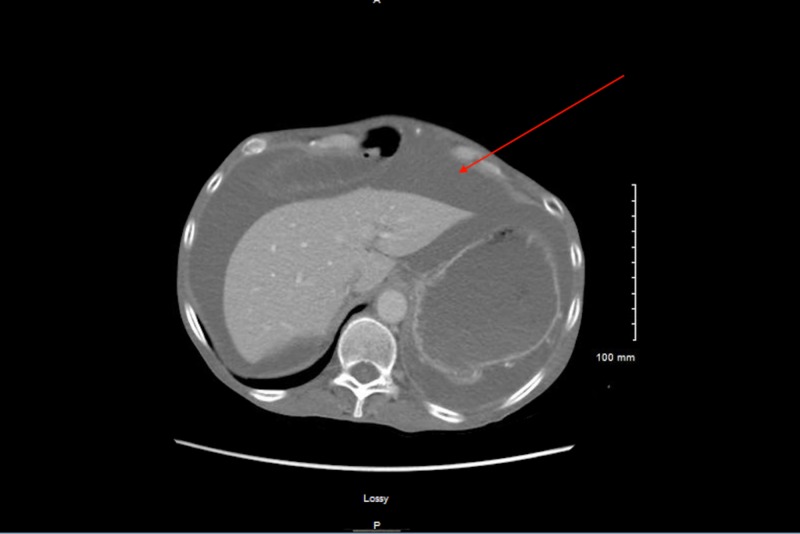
Computed tomography showing ascites
